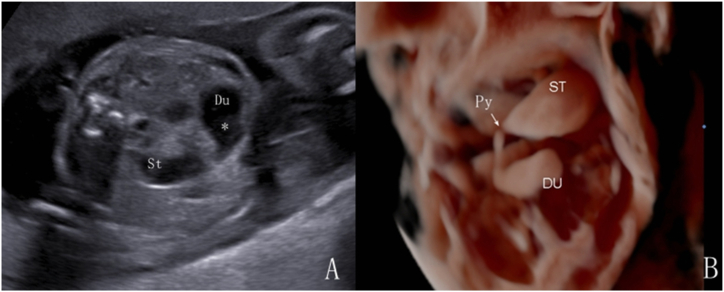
Fetus at 24 weeks of gestation with distal duodenal obstruction. Postnatal surgery confirmed horizontal duodenal atresia. A. 2D ultrasound shows the “double-bubble sign” in the upper abdomen. B. 3D volume imaging demonstrates the connection between the gastric bubble and the duodenum through the pylorus, with proximal dilation of the descending and horizontal parts of the duodenum forming a “C” shape.
